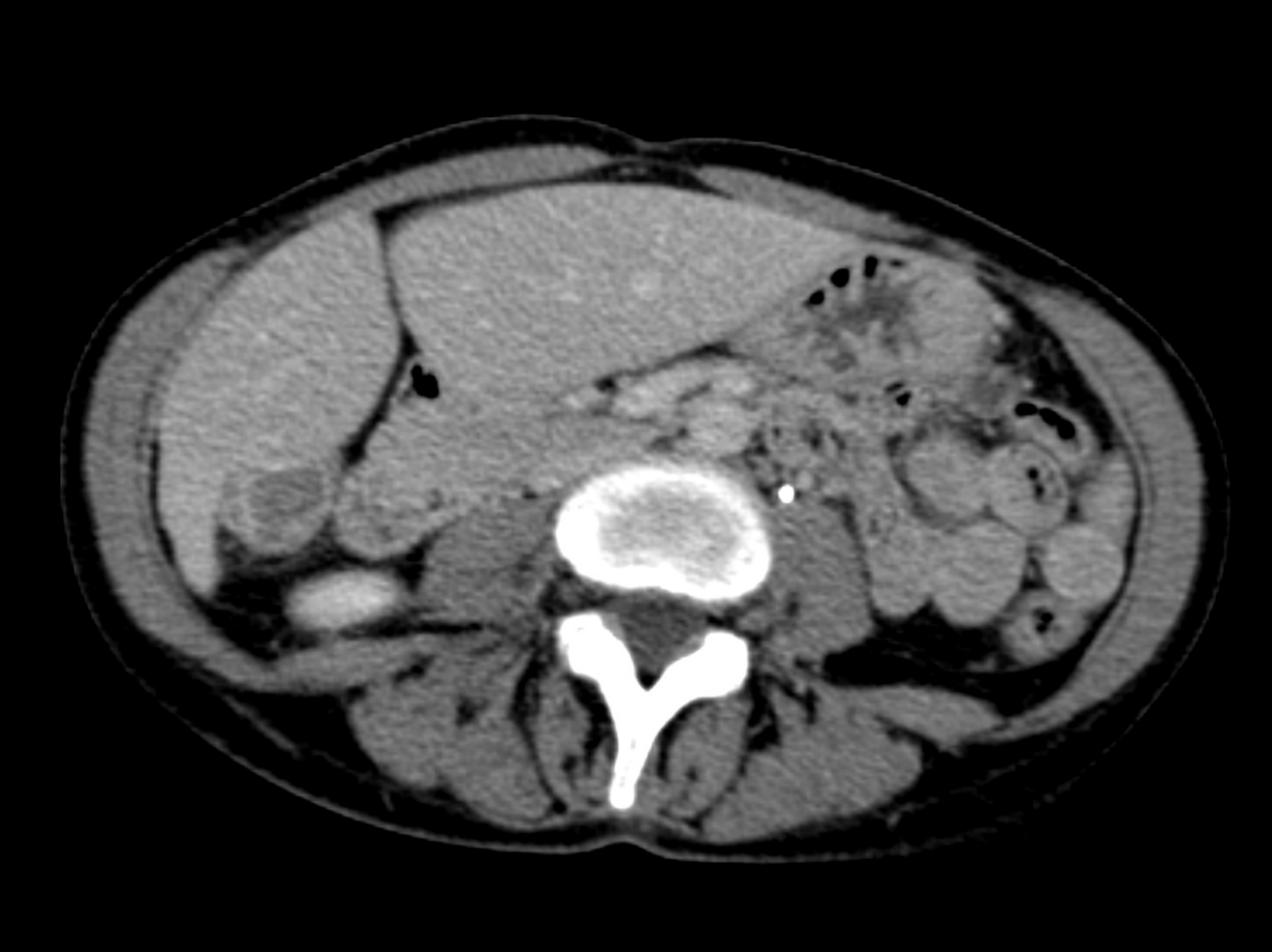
Representative axial slice from CT obtained six months after second SIRT procedure
